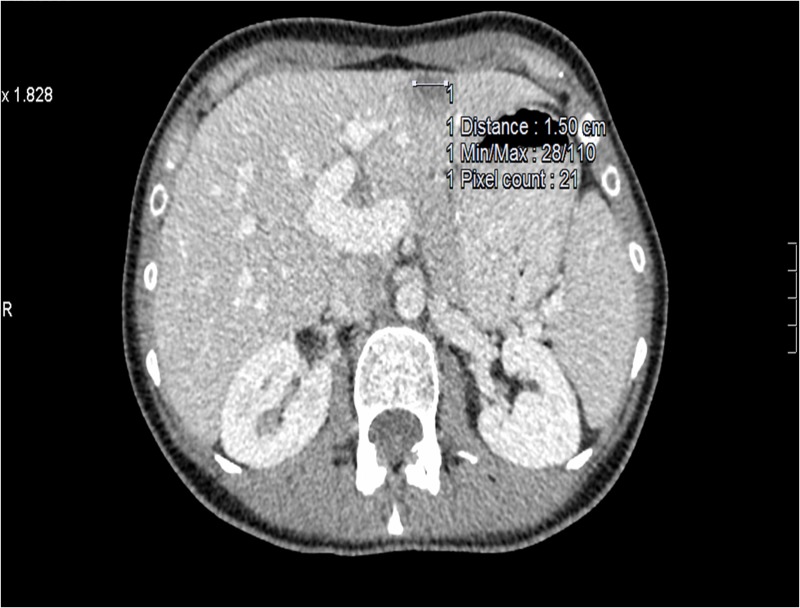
Patient 1: Baseline computed tomography (CT) at diagnosis demonstrates single liver lesion
